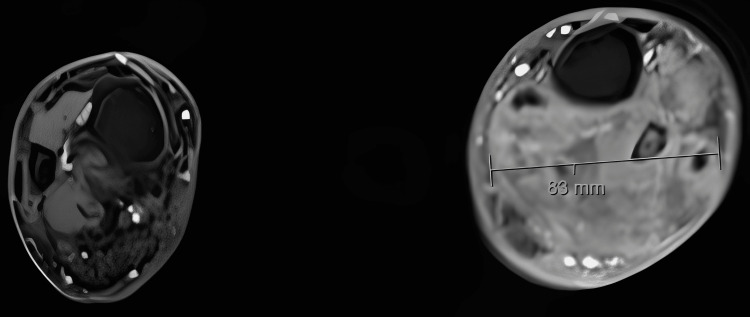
MRI revealing a soft tissue lesion involving the left fibula, measuring approximately 8 cm in transverse dimensions MRI: magnetic resonance imaging
